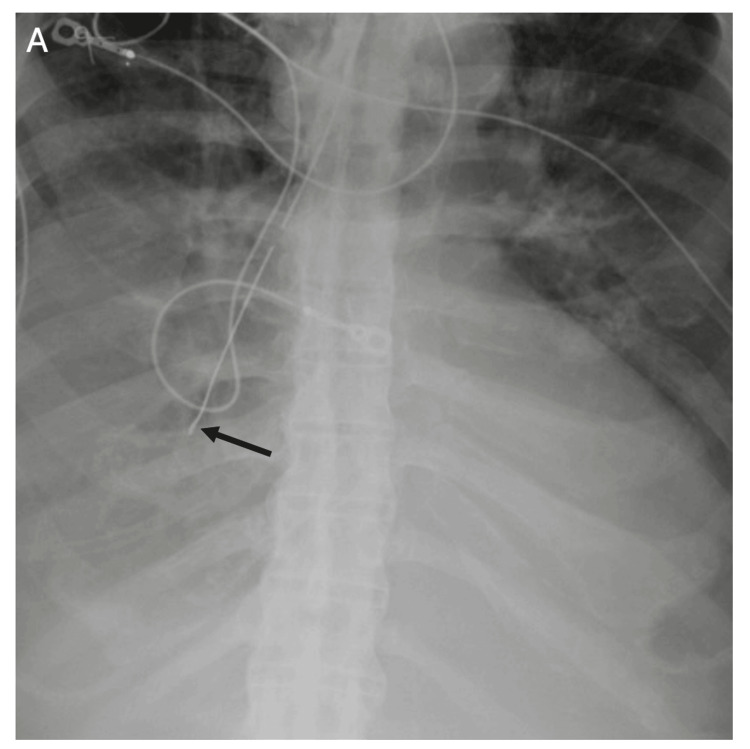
Nasogastric Tube Malposition Into the Right Main Bronchus Radiograph demonstrating malposition of a nasogastric tube into the airway. The tube is seen deviating into the right main bronchus (arrow) rather than crossing the diaphragm into the stomach. Airway malposition is more common on the right due to its more vertical orientation and carries a high risk of aspiration, pneumothorax, or feeding-related complications.
